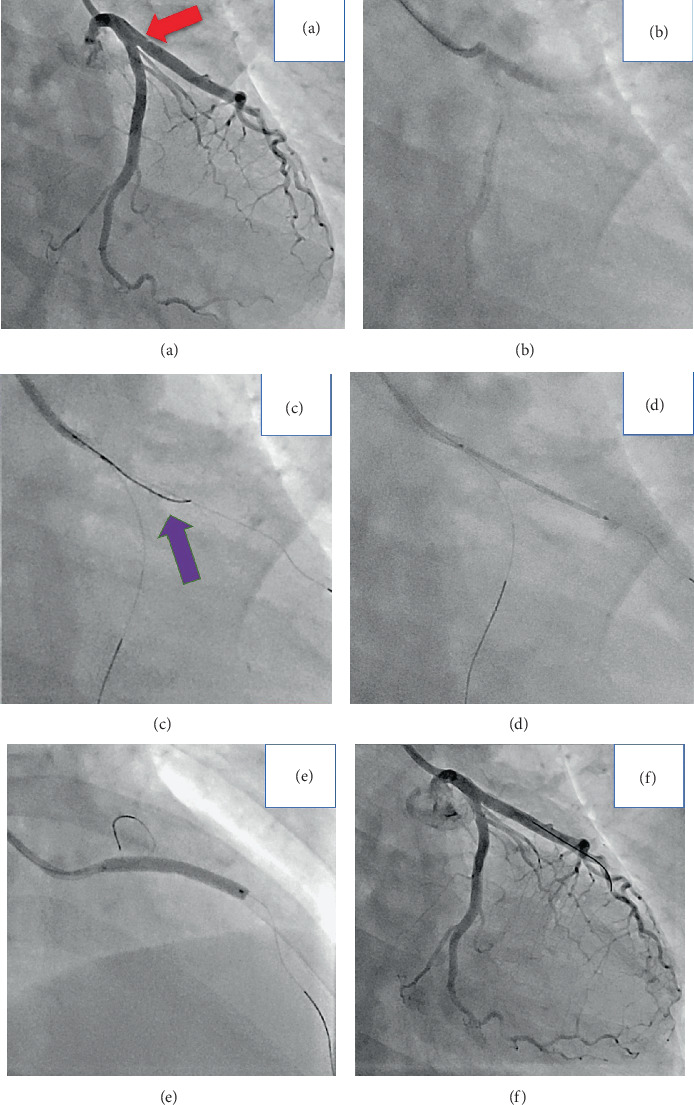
Coronary angiography and percutaneous coronary intervention. (a) Left coronary angiography (right anterior oblique caudal view) revealed a dissection at the proximal site of the LAD extending from the LMT, suggestive of type 1 spontaneous coronary artery dissection (red arrow). (b) Coronary blood flow of the LAD was impaired after coronary angiography. (c) The IVUS-guided rewiring technique was performed, and the third guide wire (purple arrow) was inserted from the true lumen of the LCX to the true lumen of the LAD by referring to the IVUS findings of the dissected segment. (d and e) A drug-eluting stent was deployed to cover the dissected segment. (f) Final coronary angiography revealed acceptable results. LMT: left main trunk; LAD: left anterior descending artery; LCX: left circumflex artery; IVUS: intravascular ultrasound.
